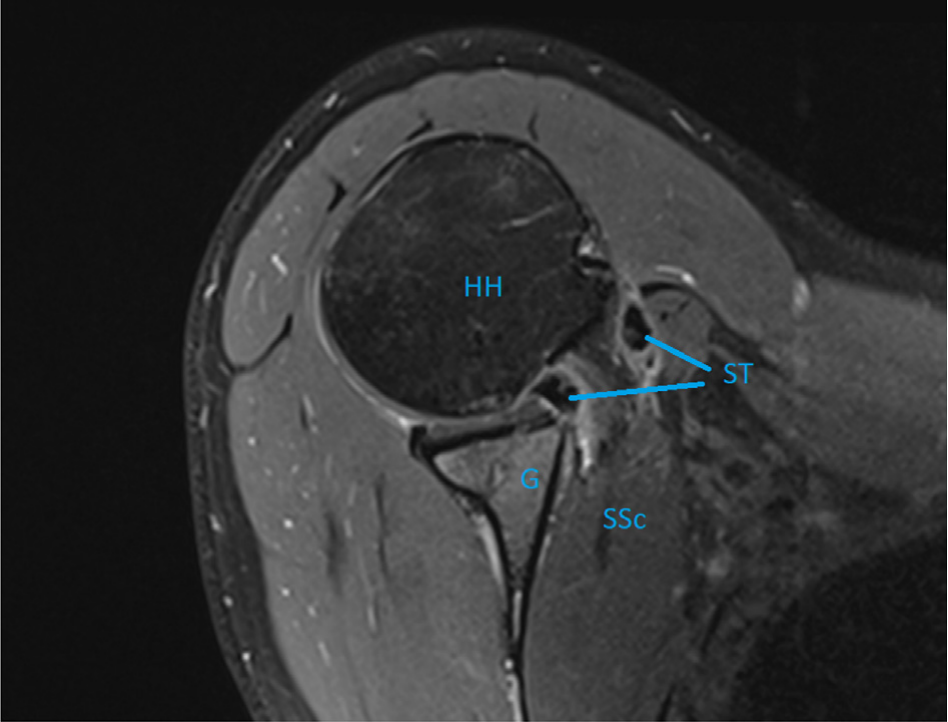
Sagittal magnetic resonance imaging of a right shoulder displaying the intact semitendinosus sling (ST) and the subscapular tendon/muscle (SSc). (G, glenoid; HH, humeral head.)
